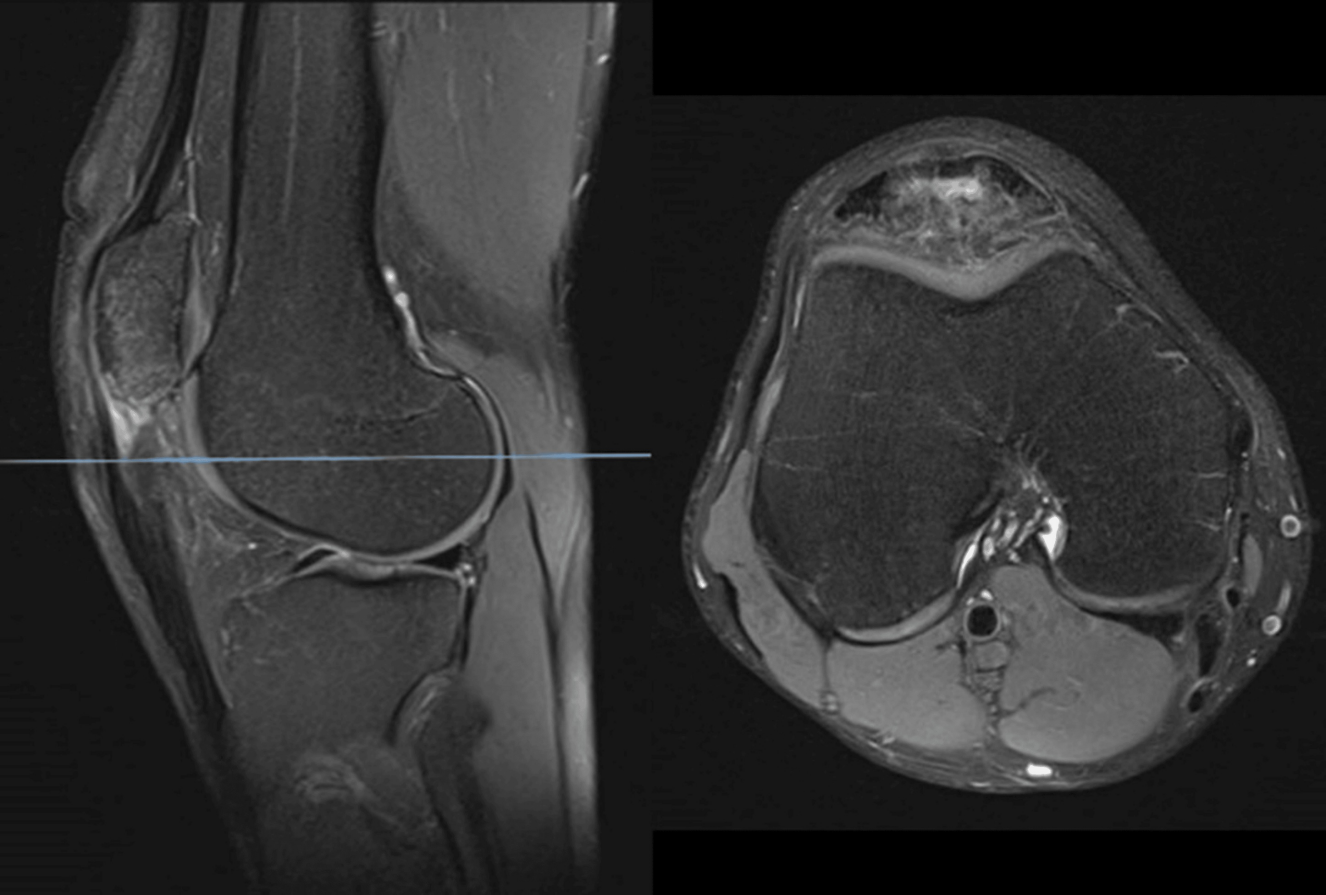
Sagittal and axial T2-weighted MRI images at first presentation, demonstrating intact extensor mechanism function. The proximal patellar tendon shows intact fibers with high signal intensity consistent with tendinopathy. MRI, magnetic resonance imaging
